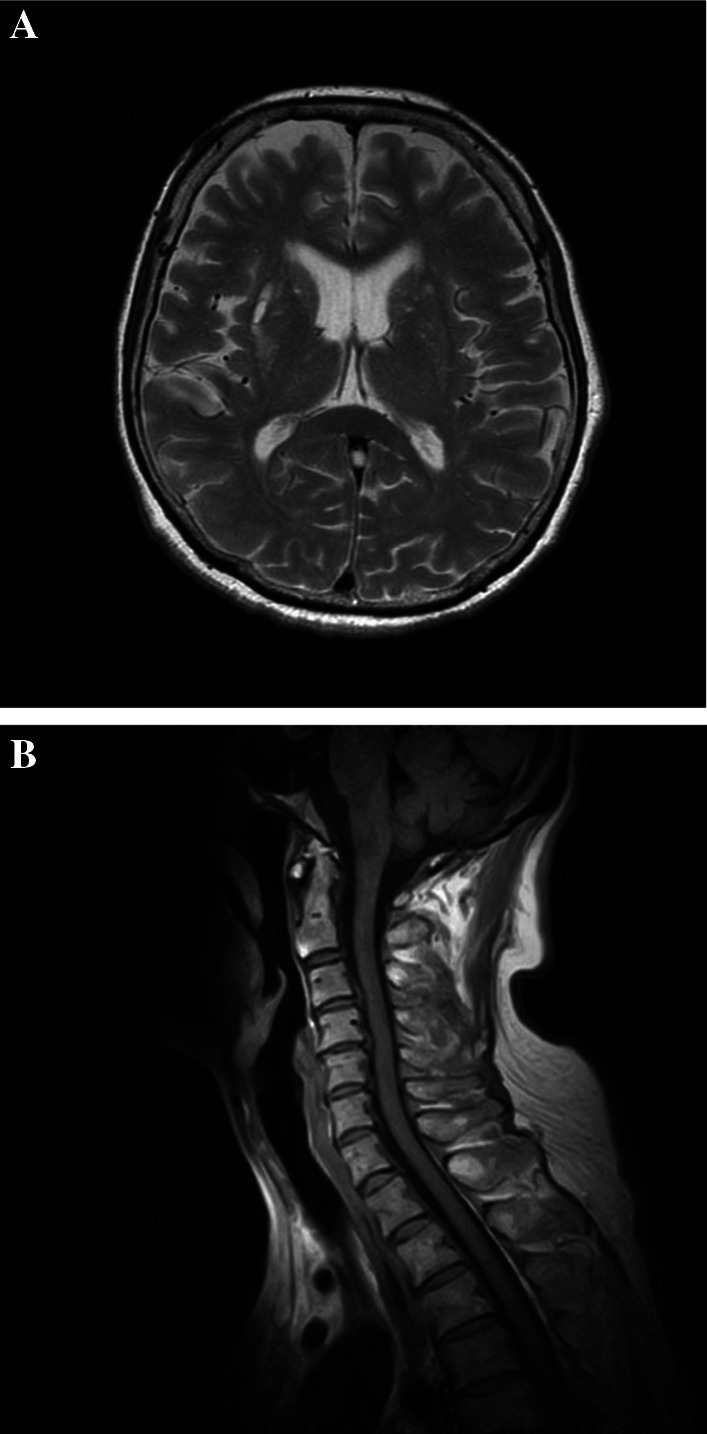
Magnetic resonance imaging. A Brain magnetic resonance imaging (MRI). Brain MRI shows multiple T2 high signals in the bilateral basal ganglia and cerebral white matter, indicating a chronic ischemic lesion. There is no evidence of brain metastasis. B Spinal MRI. C4 kyphosis and narrowing of the spinal canal of C4/5. No obvious spinal cord compression or abnormal signal is observed
